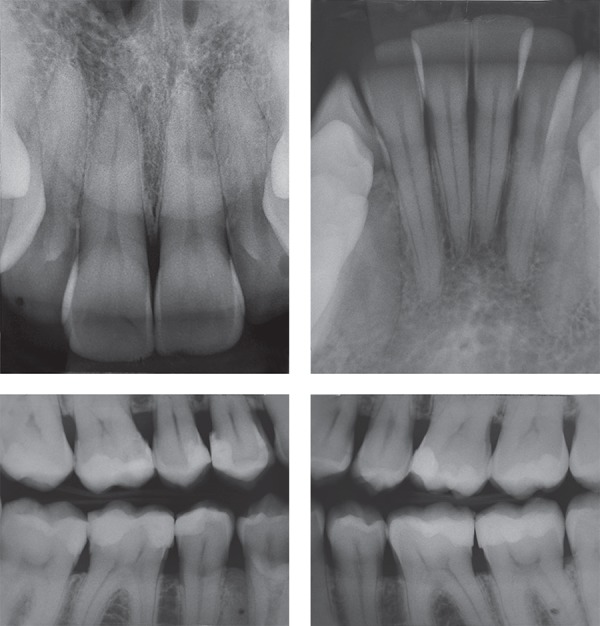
Initial periapical and interproximal radiographs of incisors.
